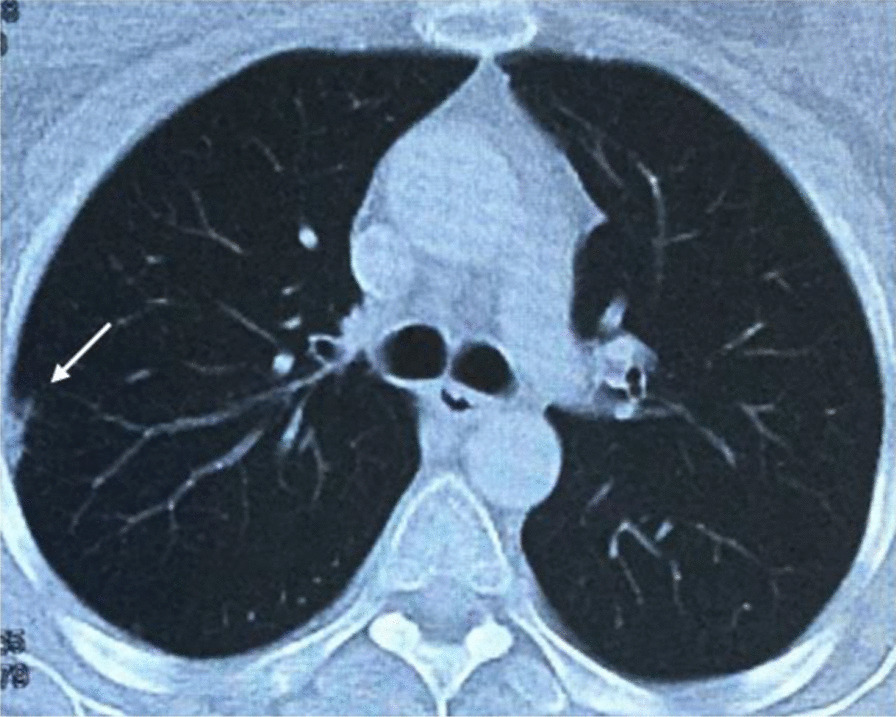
Chest CT scan showing a peripheral nodule in the lower lobe of the right lung (white arrow)
